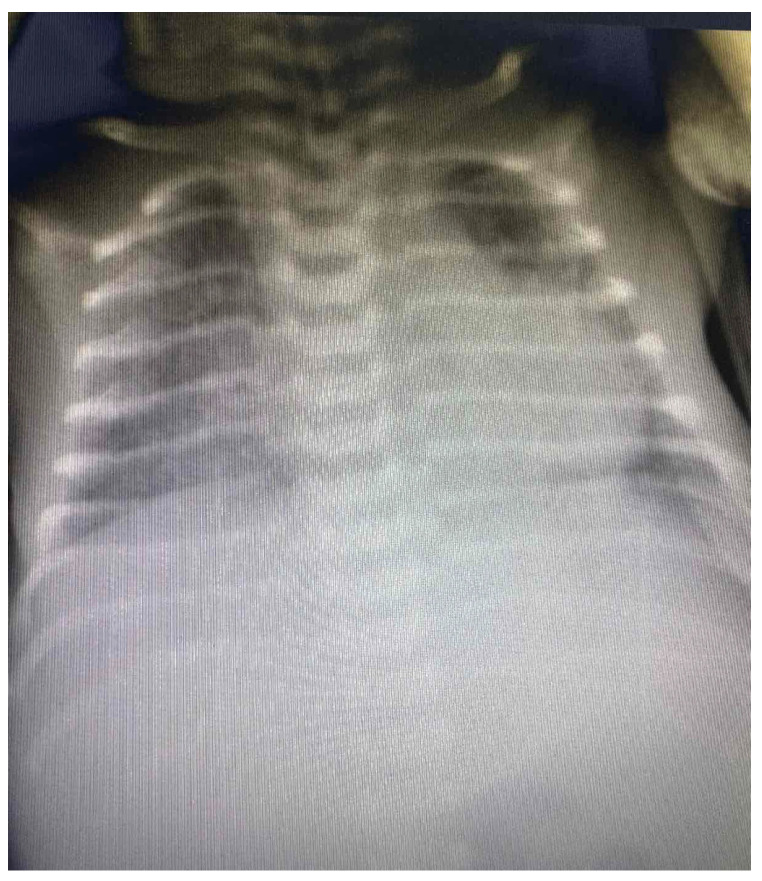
Chest X-ray revealed total opacification of the lungs, radiologically compatible with pneumonia alba.
